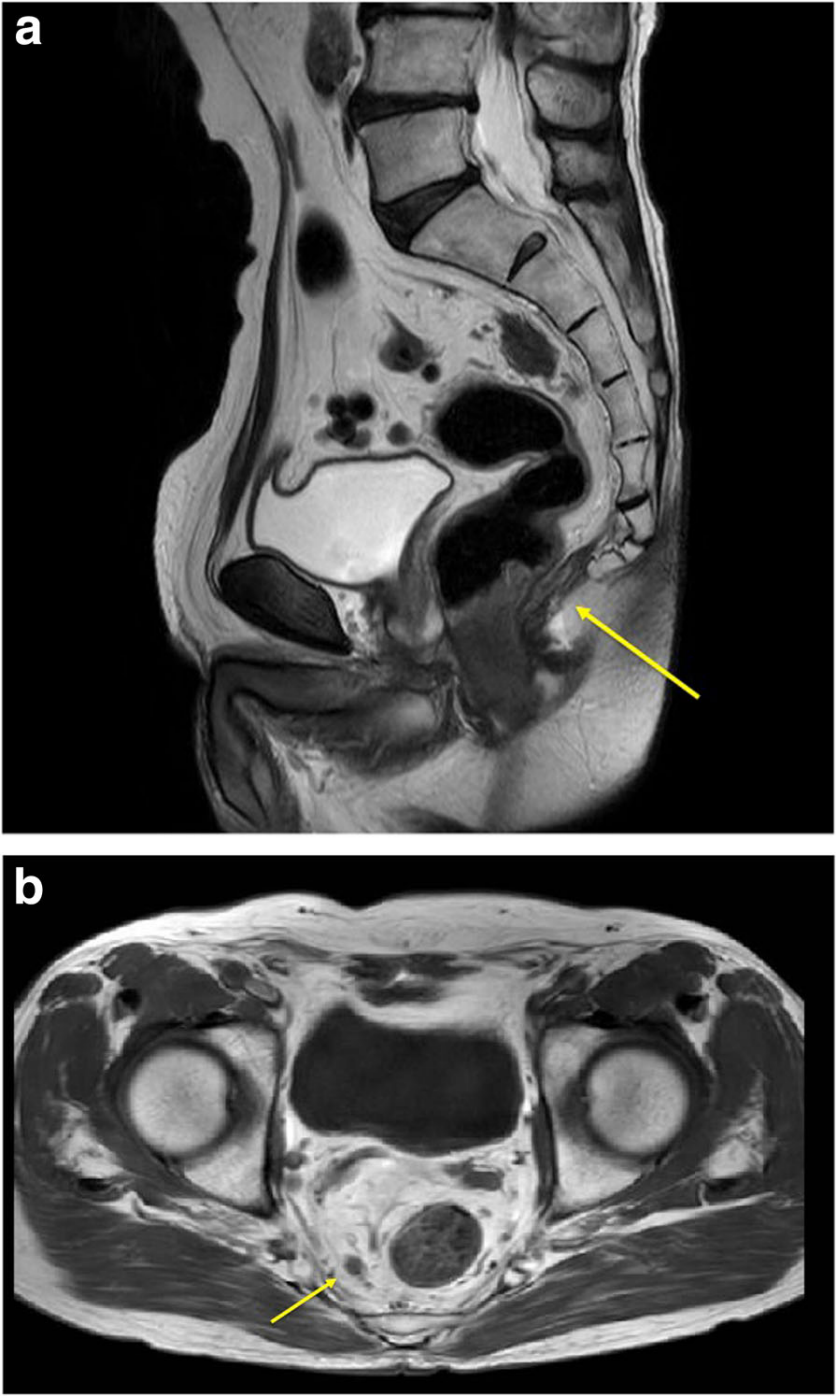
Magnetic resonance imaging. a This reveals suspected tumor invasion of the right levator ani muscle. b The right lateral lymph nodes were swollen
